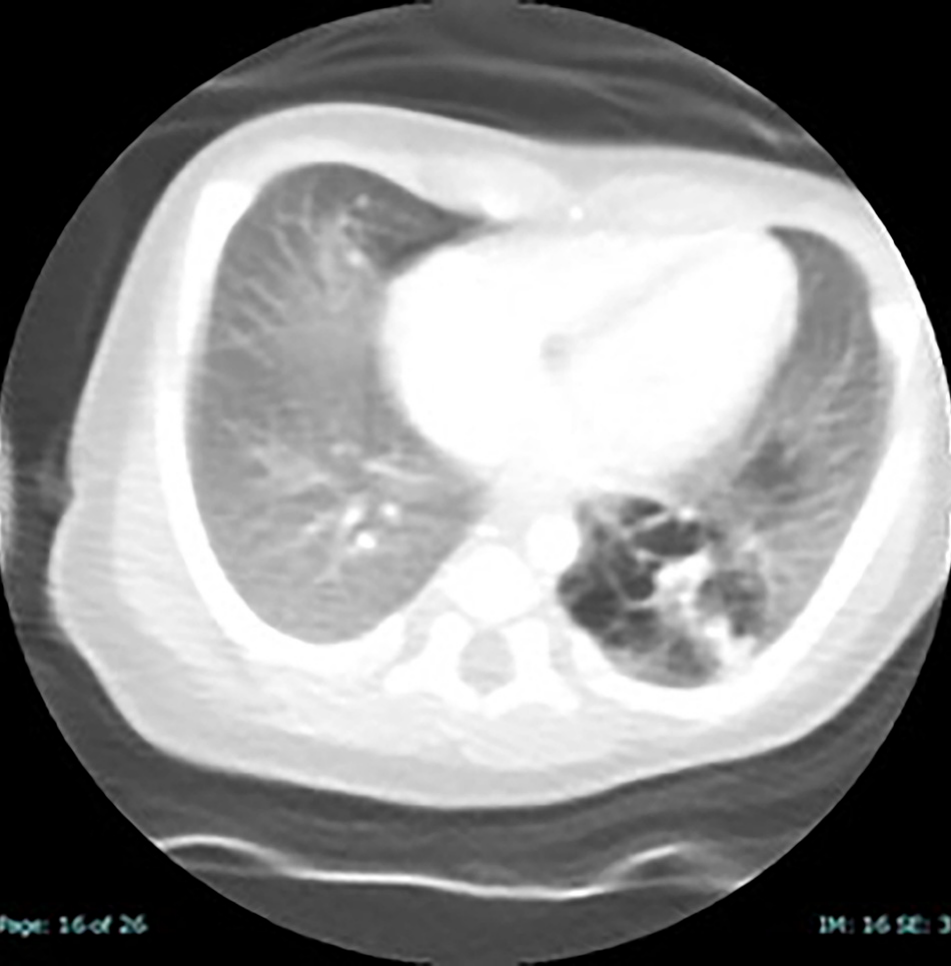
Axial CT angiogram performed on Day 47 of life demonstrating predominantly cystic air-filled CPAM hybrid lesion of the left lower lobe.
